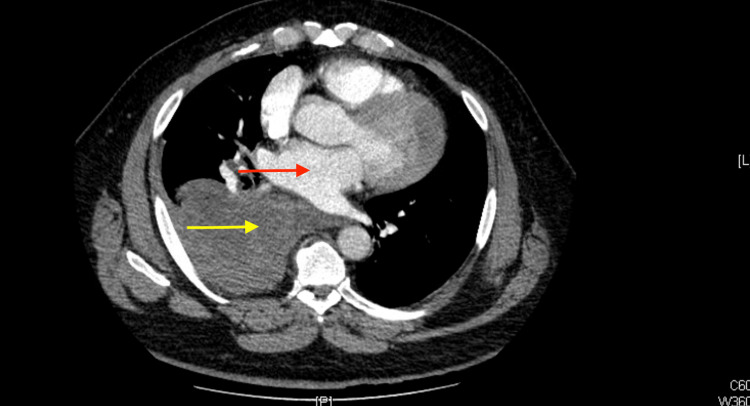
Computed tomography (CT) scan showing mediastinal mass (red arrow) with bleeding into the right pleural space (yellow arrow) resulting in hemothorax
